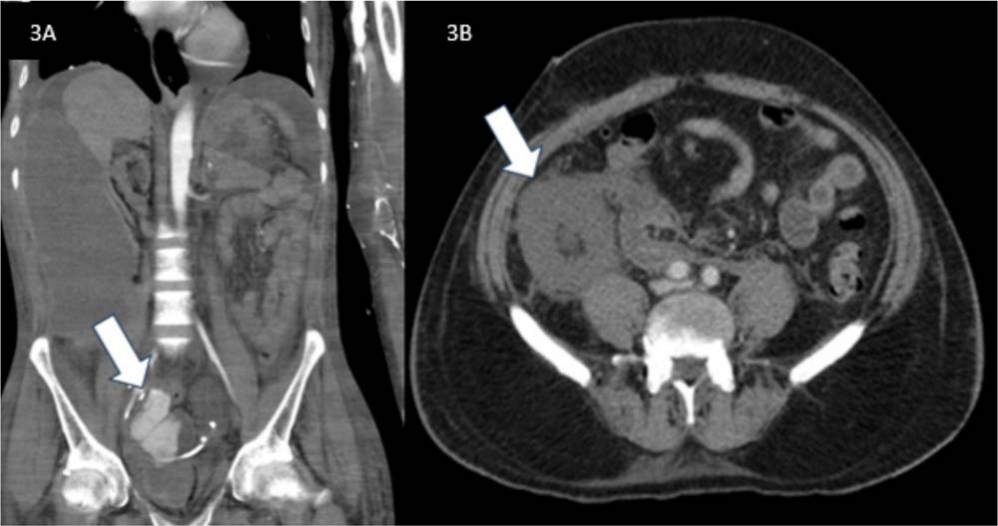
Computed tomography of the abdomen with panel 1A showing a large right abdominal hyperdense collection with active arterial extravasation (arrow) from graft anastomotic site, compressing adjacent organs in case 1 and panel 1B showing grafted kidney with no enhancement (arrow) of the parenchyma or vascular pedicle, indicating likely graft infarction in case 2.
